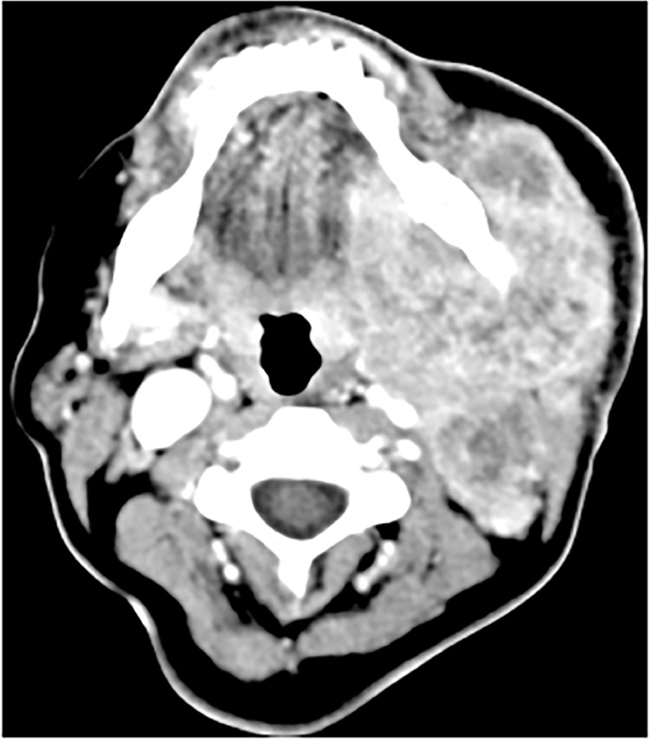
CT scan: Locally advanced left submandibular gland tumor.
